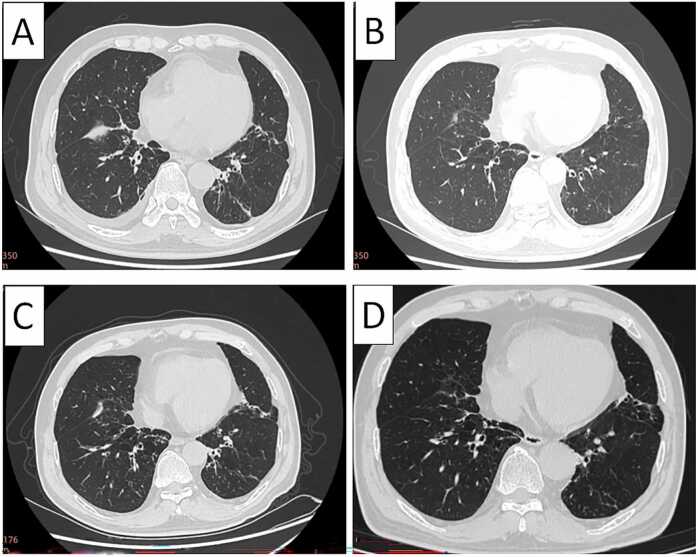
Serial chest CT images. (A) Initial presentation (August 2018): Findings at initial presentation. (B) One week post-discharge: Improvement after initial treatment. (C) Third admission: New nodule and effusion during relapse. (D) After completing 6-month therapy: Significant resolution after completed therapy.
